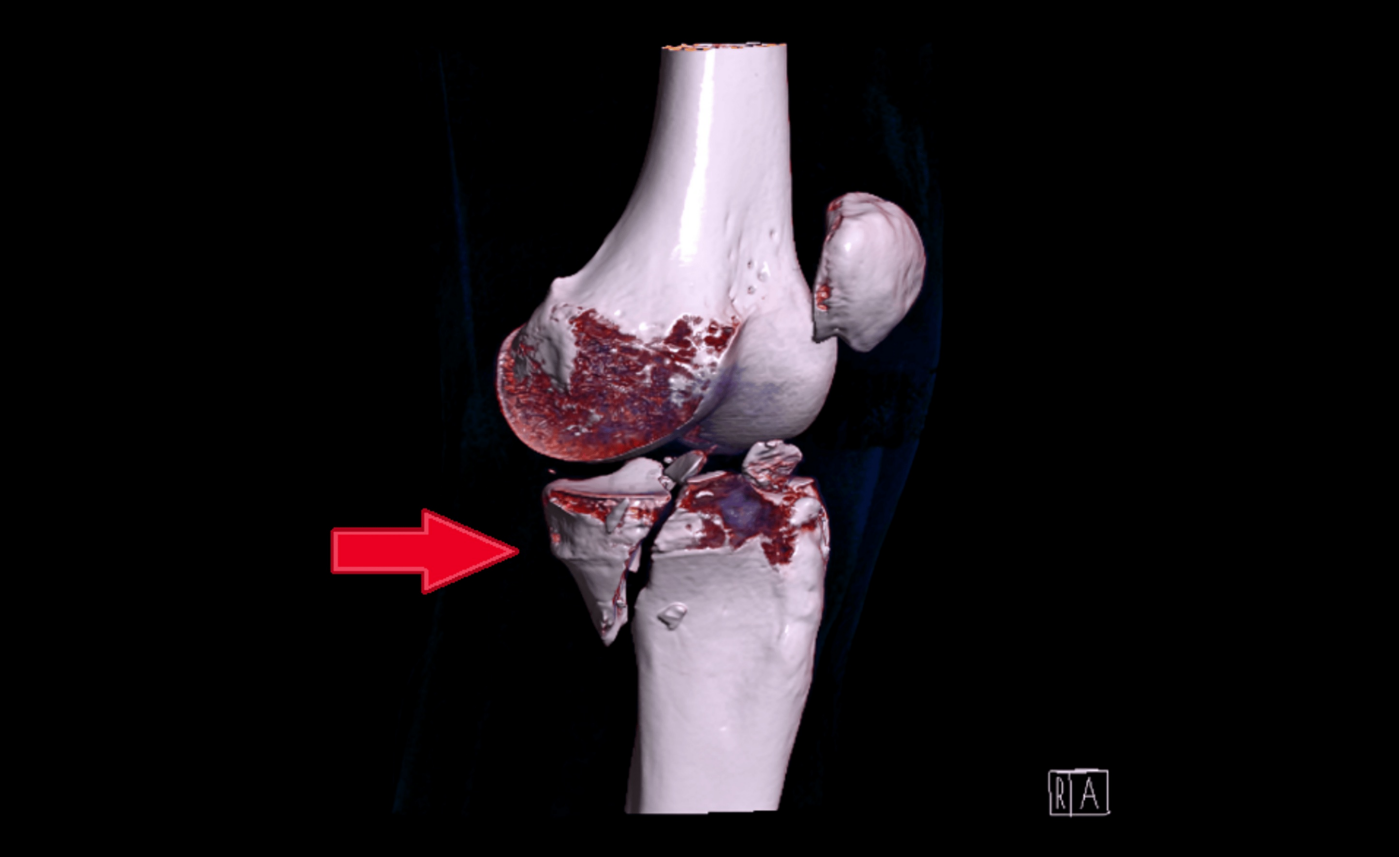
A CT scan showing a fracture of the left posteromedial tibial plateau, with the volume rendering technique (anteromedial view of the left knee)
